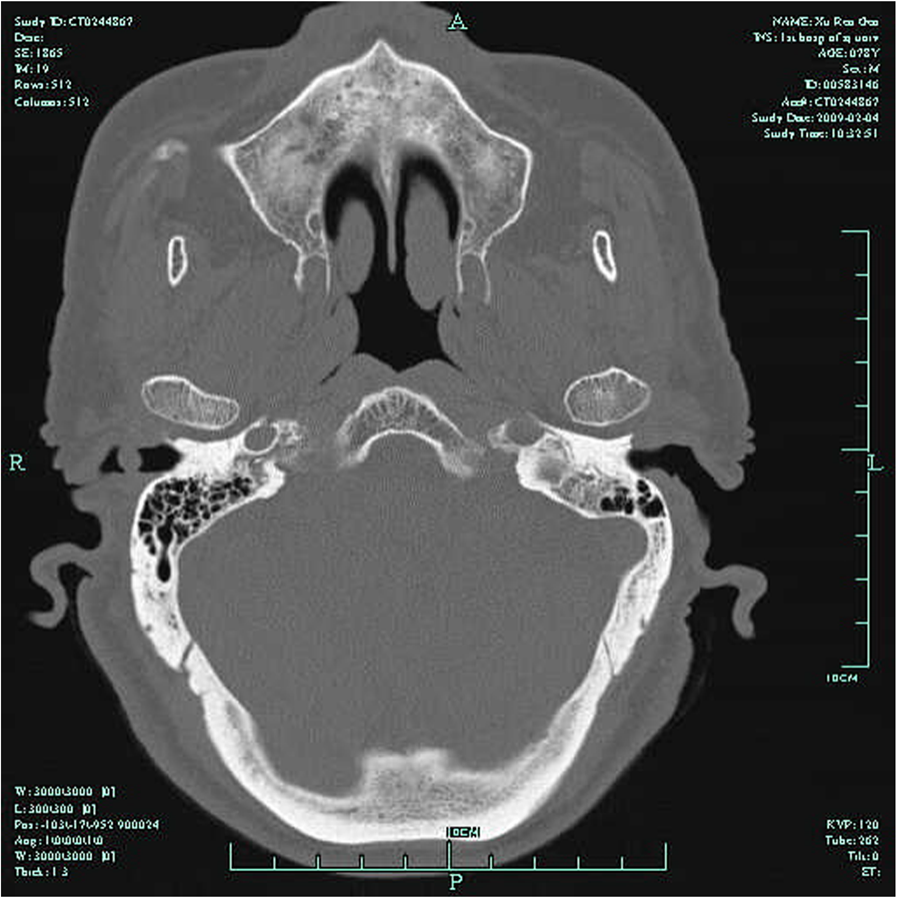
CT showed a 0.5×0.5cm soft lesion in the right EAC with no signs of bone destruction.
